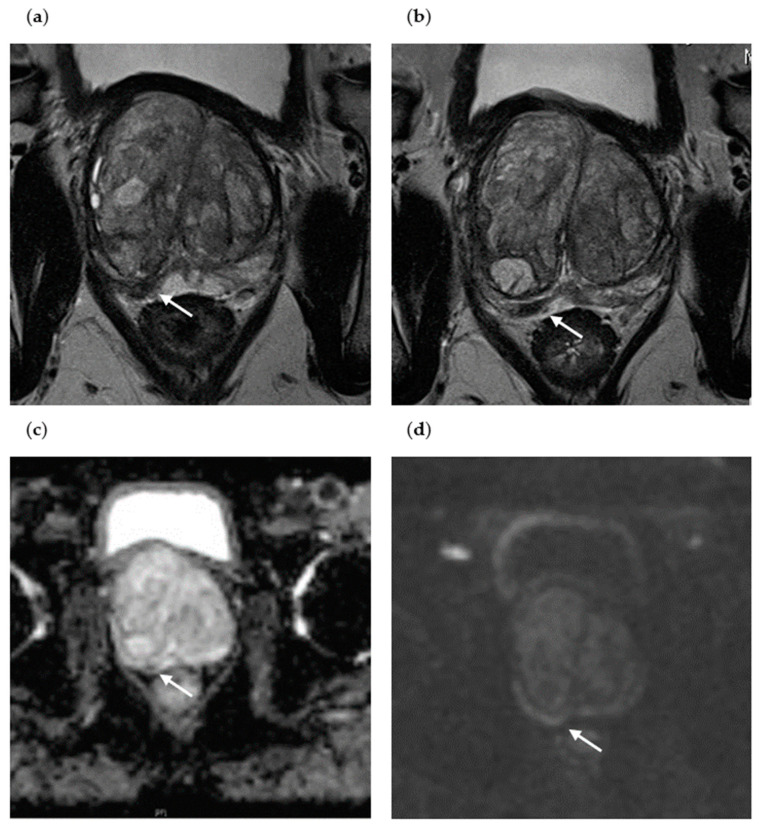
Axial mpMRI of a patient with sPC detection at repeat biopsy (Patient II). (a) Initial T2-weighted mpMRI of Patient II without PC detection at initial biopsy (PI-RADS lesion 4, the suspicious lesion is marked with an arrow); (b) repeat mpMRI of Patient II with sPC detection at repeat biopsy (persistent PI-RADS lesion 4, T2-weighted); (c) flat ADC decrease in the PI-RADS 4 lesion at repeat biopsy; (d) flat signal increase in the PI-RADS 4 lesion in the high b-value image at repeat biopsy.
